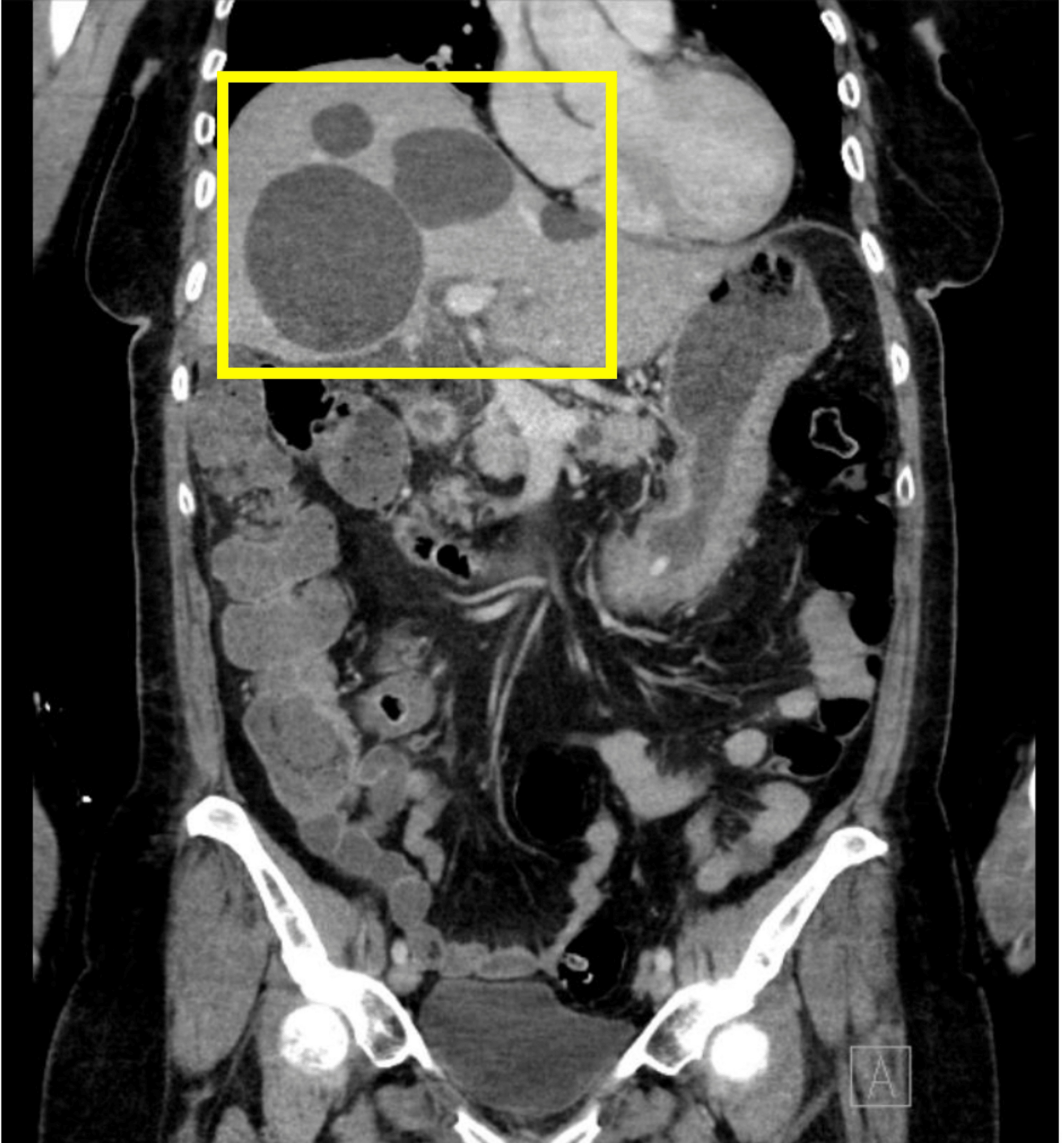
Multiple hepatic cysts labeled with a yellow square on CT scan CT: computed tomography
